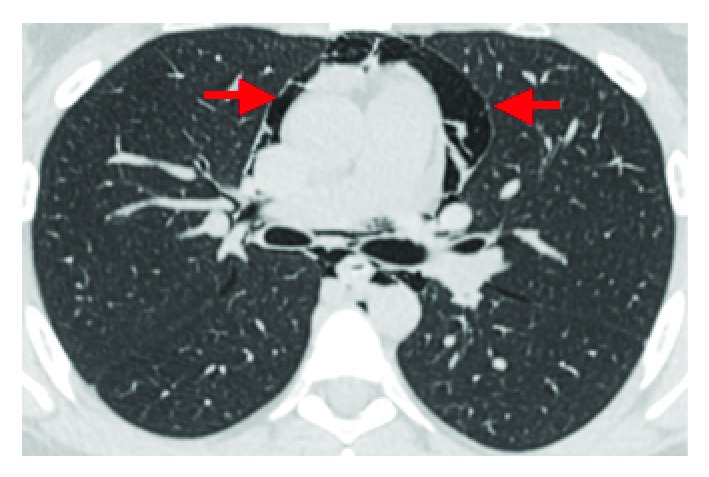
CT scan reconfirmed pneumomediastinum and subcutaneous emphysema and ruled out esophageal injury.
